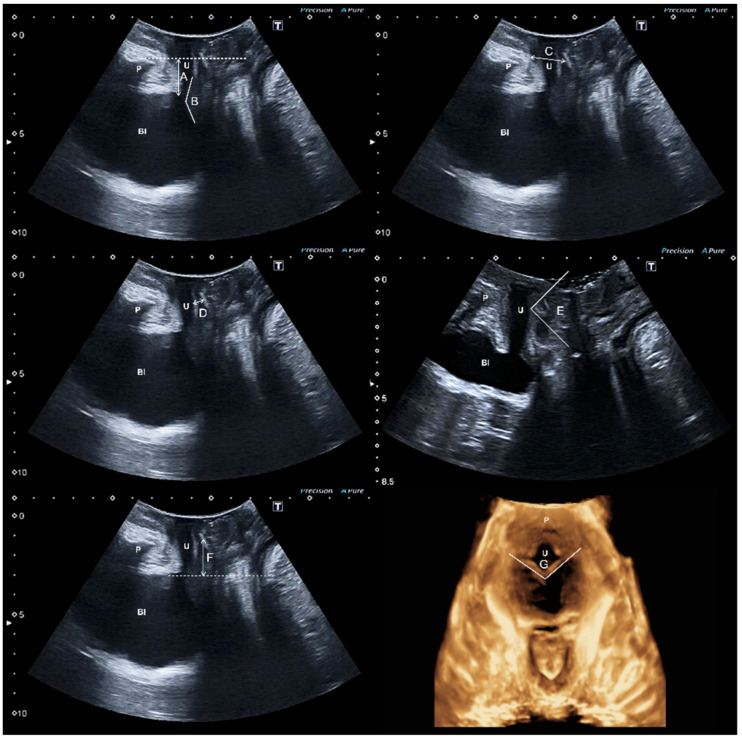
The ultrasound parameters included were the following: bladder neck–symphysis distance (A), posterior urethro–vesical angle (B), the pubic symphysis–tape gap (C), tape–urethral lumen distance (D), sagital tape angle (E), tape position (F), axial tape angle (G)m pubis (P), bladder (Bl), and urethra (U).
